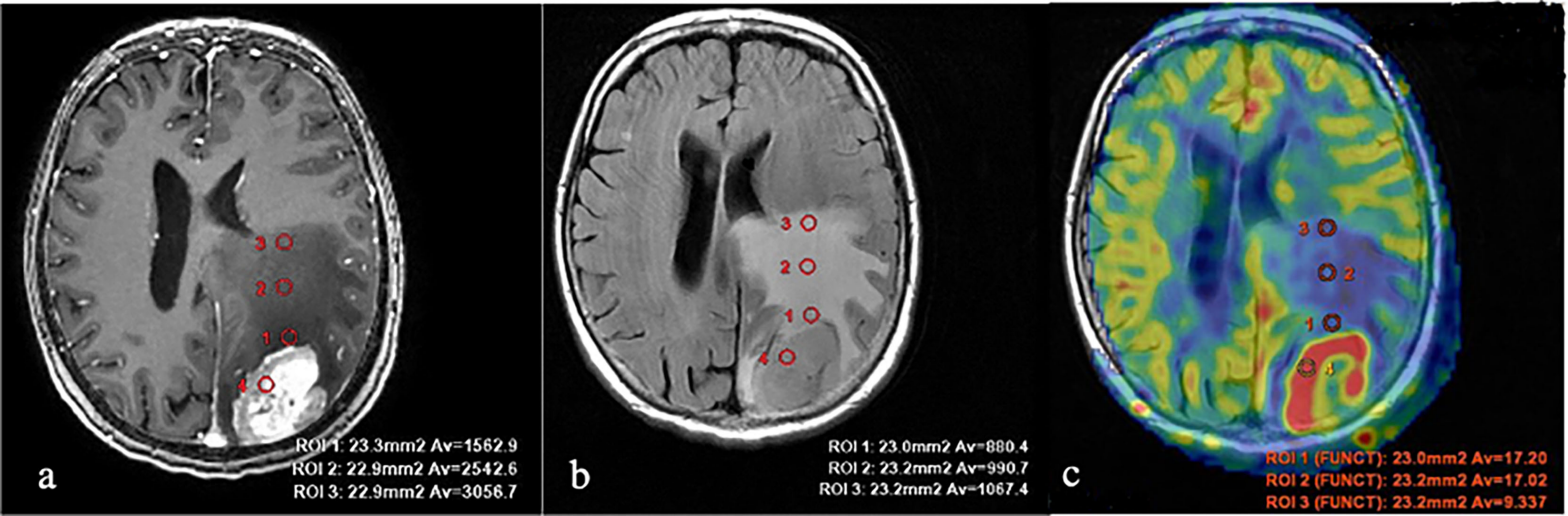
Breast cancer metastases. (A) T1 with contrast enhancement; (B) T2-FLAIR; and (C) TBF blood flow maps aligned with T2-FLAIR. Measurements of hemodynamic parameters in the tumor and peritumoral zone are shown.
